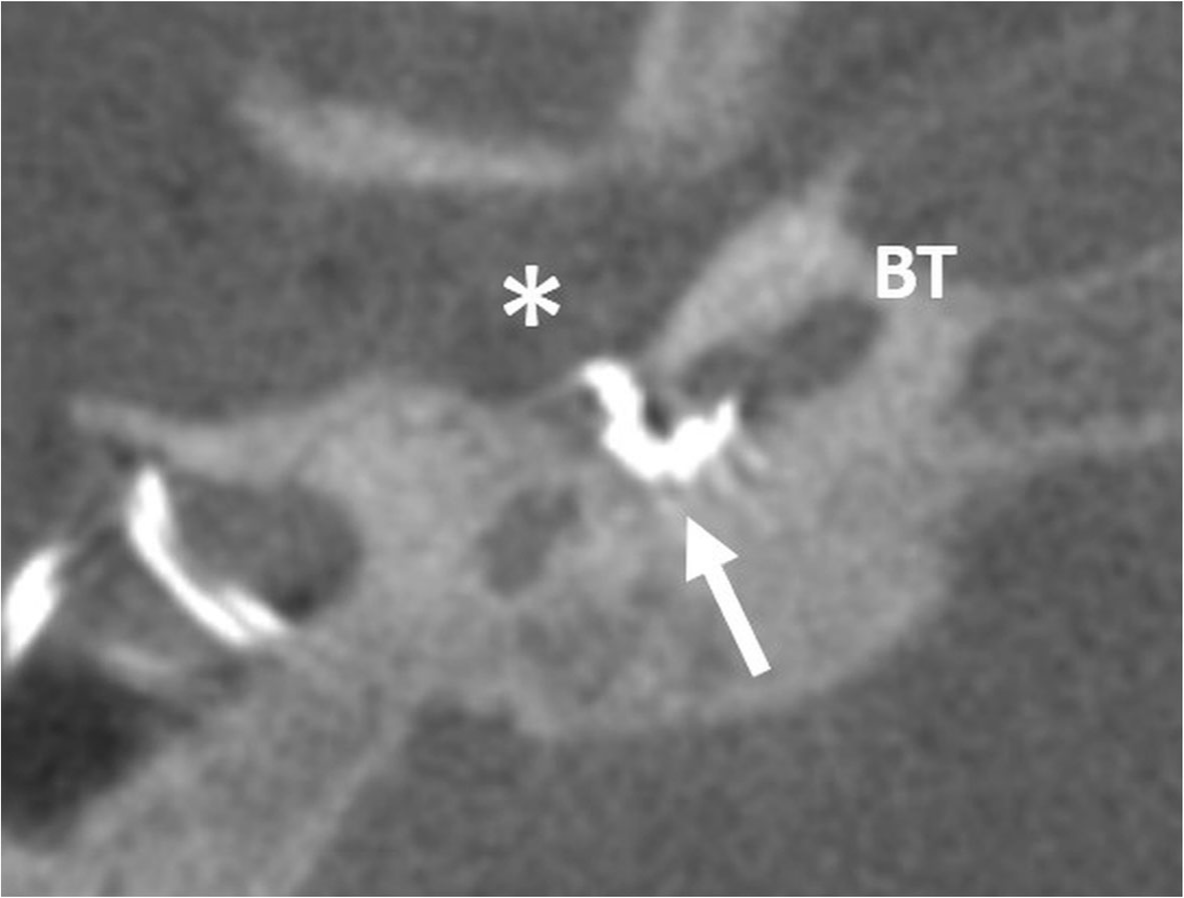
A 13-year-old female patient, with CHARGE syndrome and malposition of the electrode in the tympanic cavity (asterisk). CBCT axial image shows the apical part of the electrode array (arrow) not passing the angled entry of the round window into the basal turn (BT)
